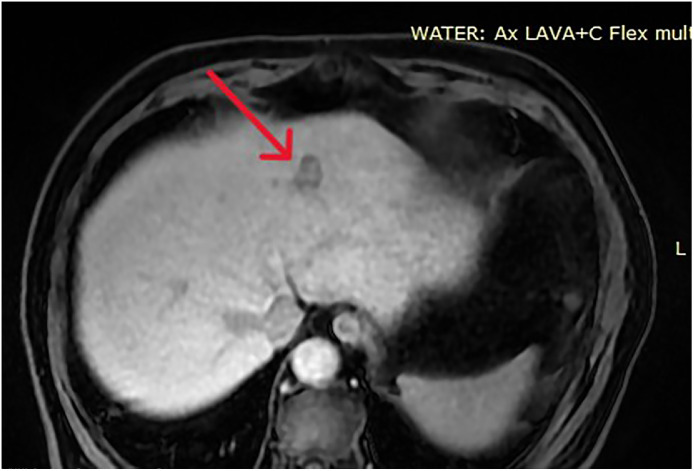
Pre-procedural MRI showing a 2.5 cm HCC in segment 2 (red arrow) with a close anatomical relationship to the pericardium.•The patient underwent MWA under general anesthesia using combined CT and real-time ultrasound (US) guidance. MWA was selected over Radiofrequency Ablation (RFA) due to its faster heating cycles and reduced susceptibility to the 'heat sink effect' from adjacent large hepatic veins, ensuring a more predictable ablation zone in the cirrhotic liver. During planning, an 8 mm bridge of healthy hepatic parenchyma was identified between the tumor margin and the diaphragm/pericardium. Consequently, hydrodissection was not performed as the distance was deemed safe according to standard protocols.•The MWA antenna was positioned under real-time US and CT guidance (Fig. 2 (A), Fig. 2 (B)). Toward the end of the ablation cycle, the patient experienced sudden hemodynamic instability: profound hypotension, tachycardia, and facial cyanosis. While ultrasound initially identified a pericardial effusion, an immediate emergency CT was performed to exclude other life-threatening complications, such as active parenchymal bleeding or pneumothorax, and to provide precise anatomical mapping for the subsequent drainage (Fig. 3A).
